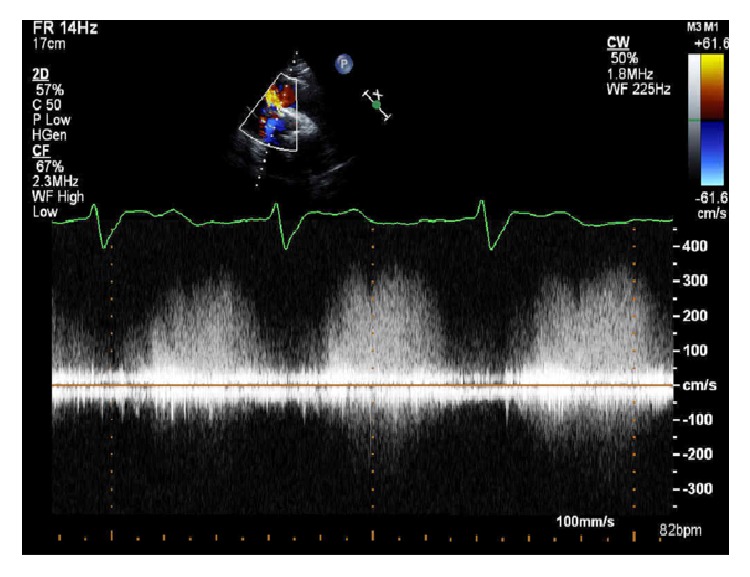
1-Month follow-up: TTE 2D ECHO; this shows continuous wave Doppler across the shunt between the aorta and RV.
